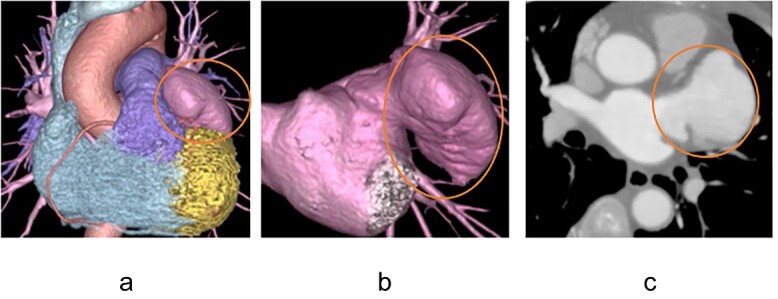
Preoperative contrast-enhanced computed tomography image A large left atrial appendage aneurysm was found, but no internal thrombus is visible.
